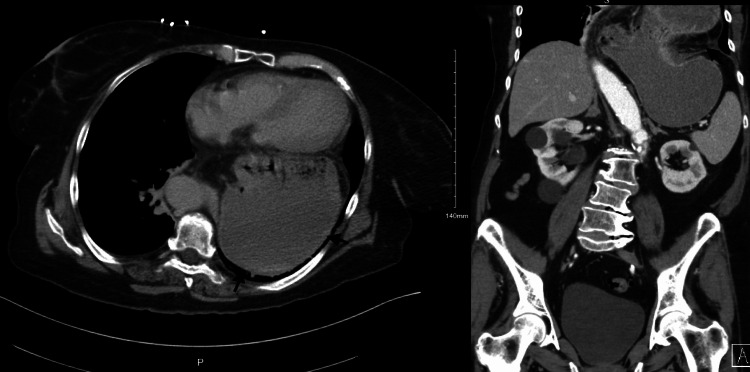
Left: Axial CT image shows the retrocardiac position of the stomach. Right: Coronal reformatted CT images show a large diaphragmatic defect with portions of the stomach within the thoracic cavity
